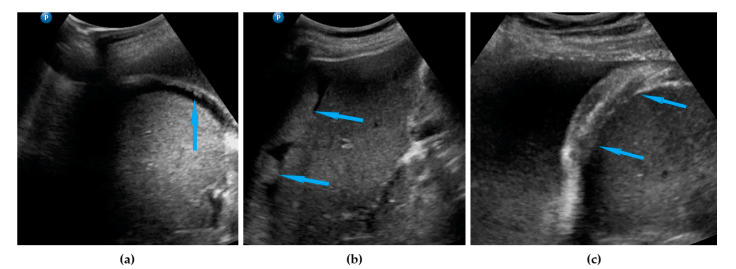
Ultrasound images of right diaphragm peritoneum involvement: (a) nodules; (b) tumors; (c) diaphragm thickening. Blue arrows identify lesions.
